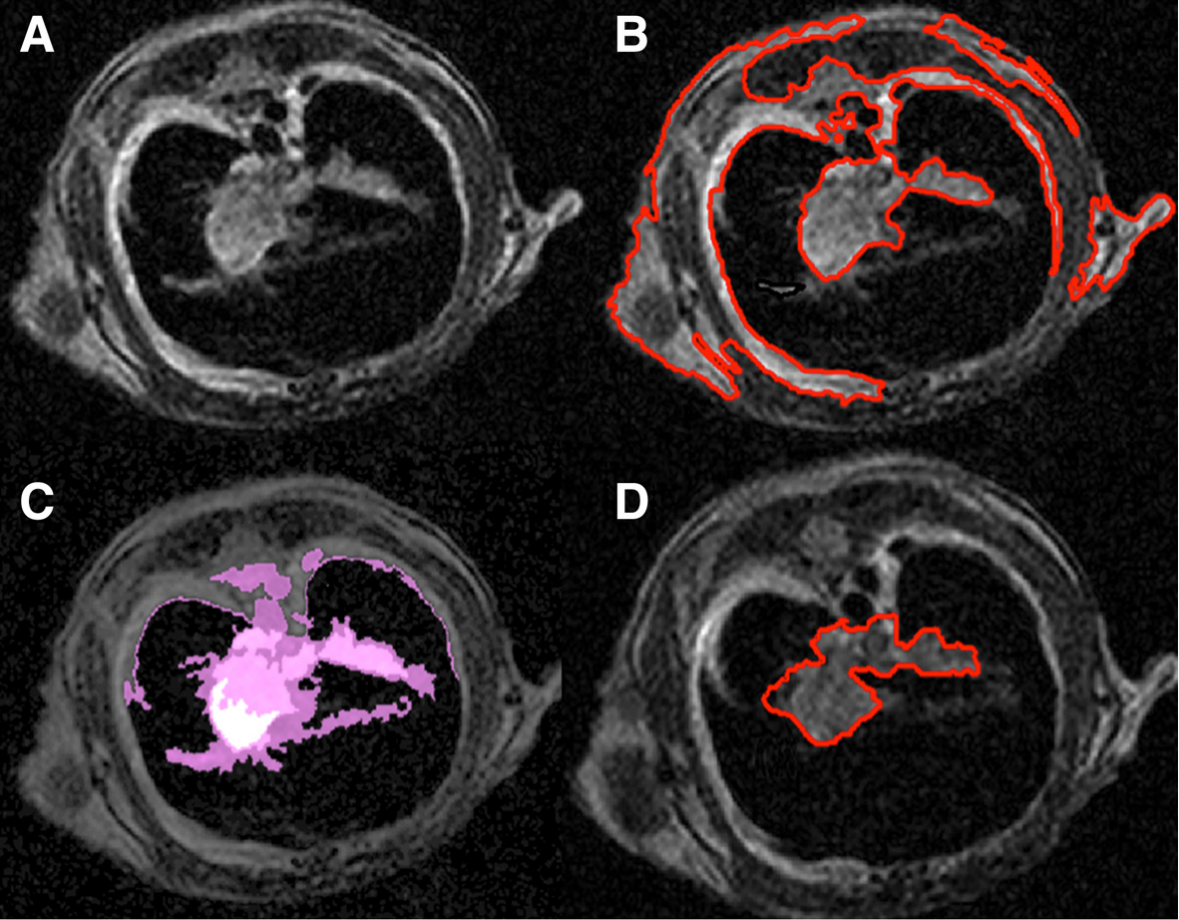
Segmentation evaluation through comparison to the state-of-the-art methods. For a given MRI slice (A), a qualitative comparison of the proposed segmentation (D), with respect to two of the state-of-the-art segmentations (i.e., region growing in (B) and graph cut in (C)), is shown. Boundary of the tumors in (B) and (D) are shown in red. Regions identified by graph cut (C) are shown in pink.
